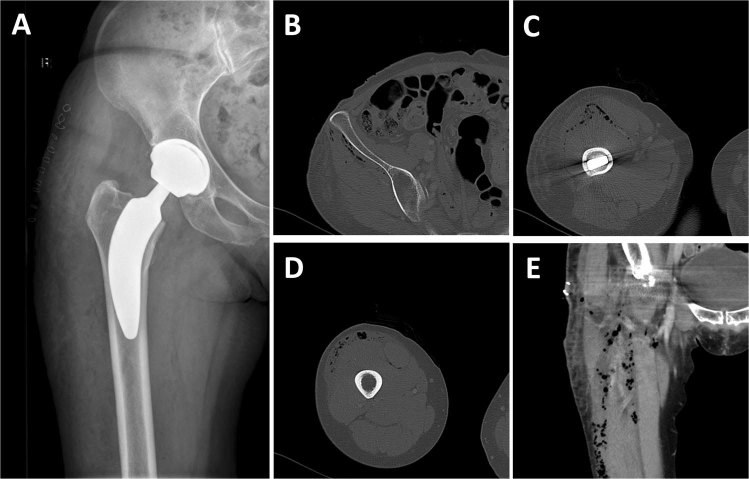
Clinical case. X-ray taken directly postoperatively from a 57-year-old female patient who had undergone THA (ANA NOVA Alpha 50/44/36 M/Proxy 8) of the right hip. (A) X-ray of the left hip taken 1 day postoperatively (B) and corresponding CT-scan (C). Note the air distribution from the anterior superior iliac spine (B) down to the proximal 1/3 (C) and mid 1/3 of the femur (D,E).
